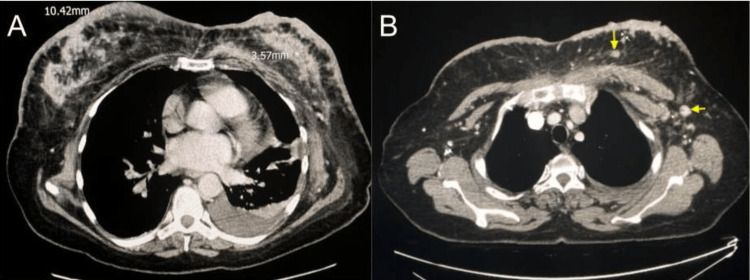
CECT of the chest (axial view). (A) Diffuse irregular skin thickening with dermal lymphatic extension and axillary adenopathy, asymmetric bilateral heterogeneously enhancing breast masses with spiculated borders and subtle surrounding fatty stranding, and innumerable metastatic nodules with the replacement of normal fatty hilum that conform to the fibroglandular tissue and axillary nodal stations. There is a central dystrophic calcification within the left breast mass with adjacent infiltration along the anterior chest wall, reticular thickening of the pectoralis musculature, and a malignant left pleural effusion. (B) Enhancing axillary adenopathy, level 1 and level 2 nodes on the left. CECT: contrast-enhanced computed tomography
